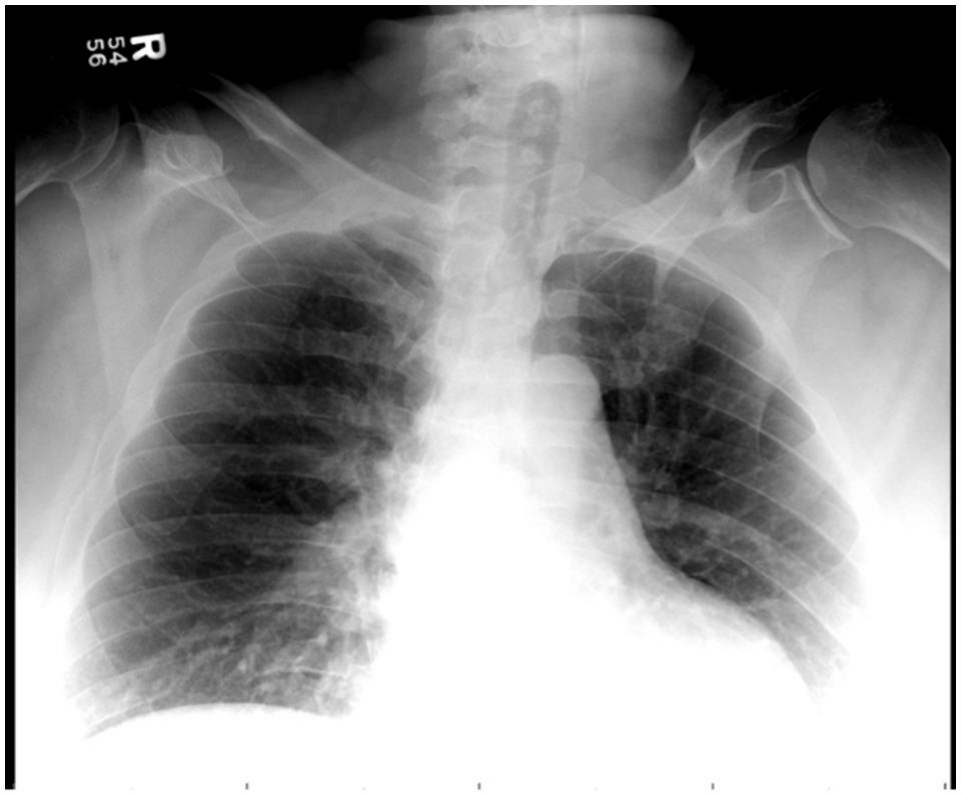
Radiograph of the chest obtained during the first few days of symptom onset appears normal. However, a normal radiograph cannot exclude potentially life-threatening pathology. Additional imaging should be obtained when there is a clinical concern for osteomyelitis and/or necrotizing fasciitis.
